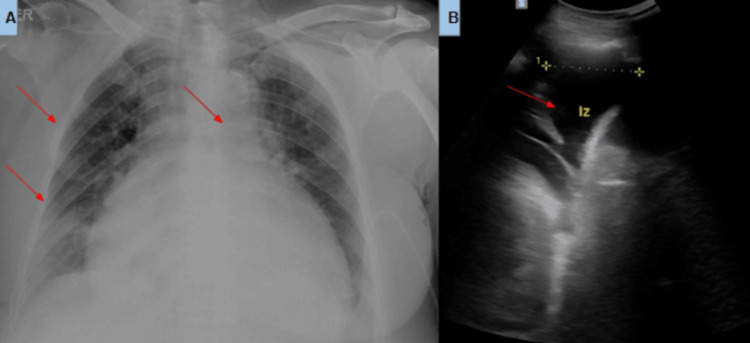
(A) Anteroposterior chest X-ray demonstrating cotton-wool infiltrates (arrows), cardiomegaly, and bilateral pericardial and pleural effusions. (B) Lung ultrasound image showing a large, 1000 mL left pleural effusion (arrow)
